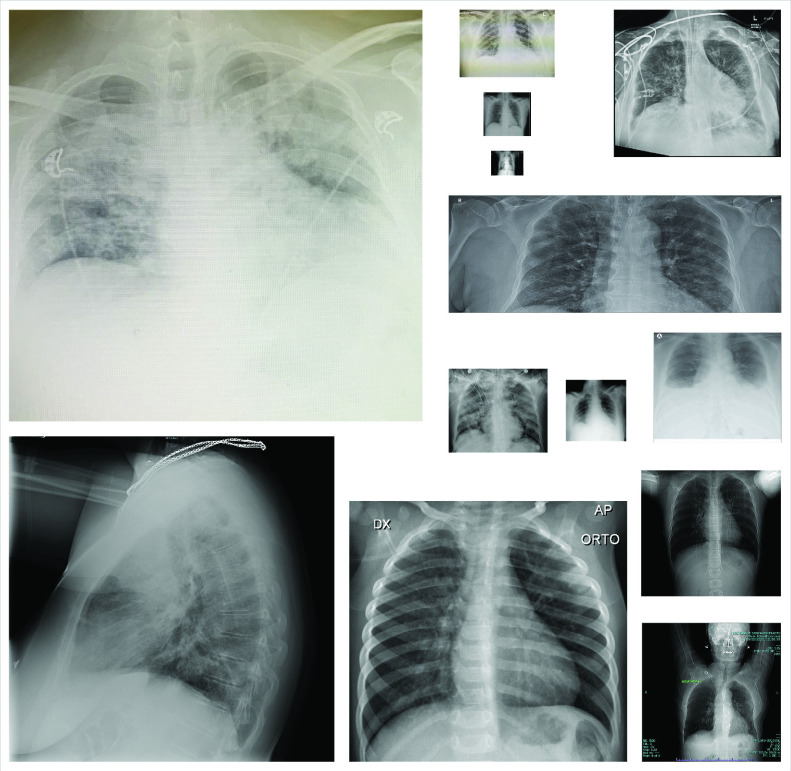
Sample COVID-19 X-ray images from QaTa-Cov19.
